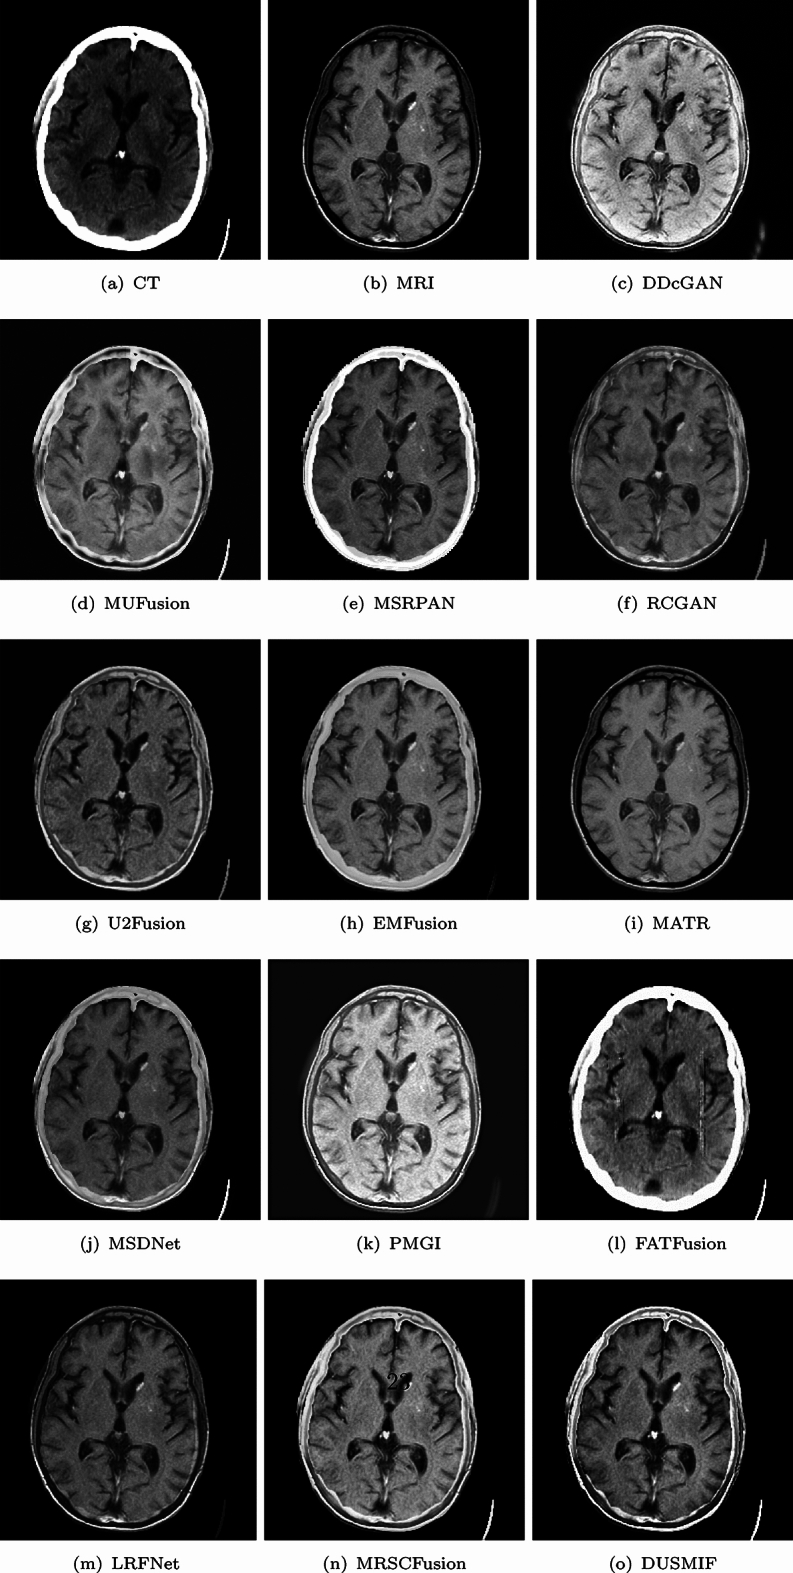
The first comparative display of CT-MRI medical image fusion.
